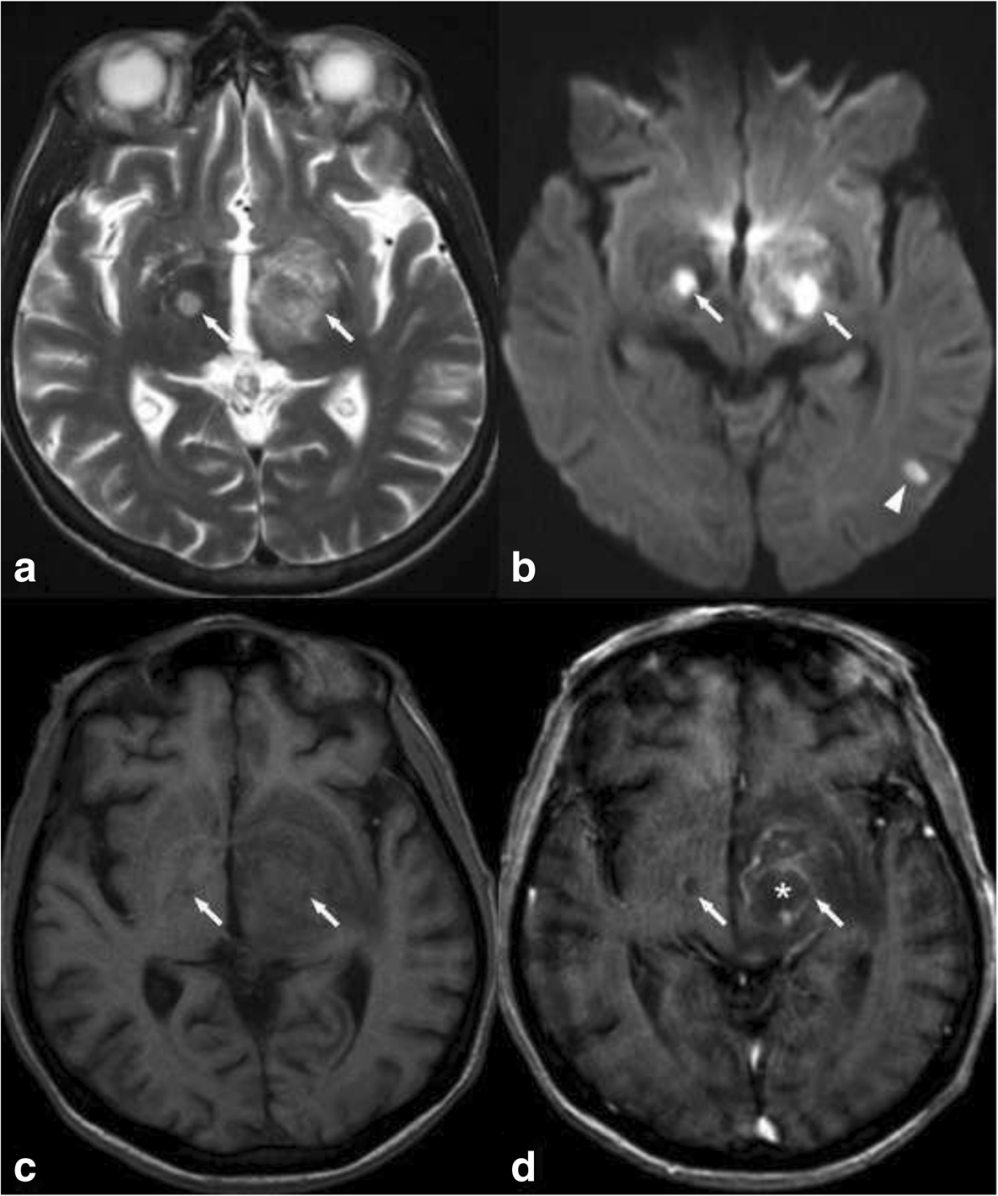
T2WI and DWI hyperintense lesions in both thalami (arrows in a and b). The lesions are hypointense on T1, and there is a non-enhancing central region corresponding to an area of necrosis (* in d). This pattern of enhancement suggests lymphoma in immunocompromised patients
